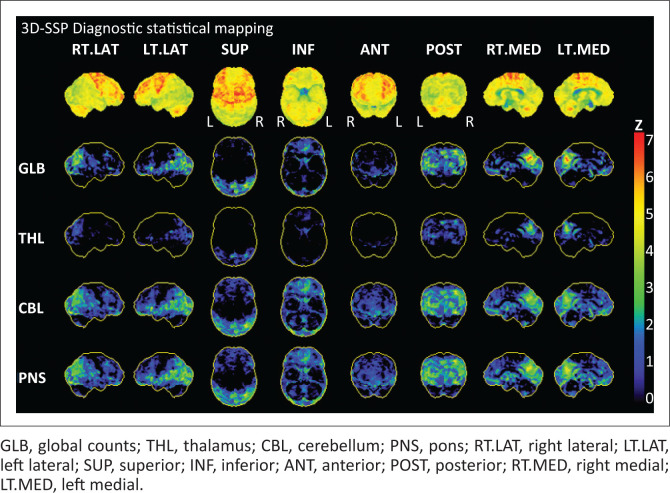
Example of a single-subject statistical analysis (decreases) for a FDG PET scan of a patient with dementia with Lewy Bodies. The top row represents surface projections of both brain hemispheres from different angles. Subsequent rows represent negative deviations from the normal database mean (z-score) for all brain regions, normalised to global counts (GLB), thalamus (THL), cerebellum (CBL), and pons (PNS). Similar outputs are generated for statistical *increases* (not shown). In this example, reduced metabolism in bilateral parietal occipital vortices is evident. Such analyses are highly recommended to support the interpretation of brain PETs.
